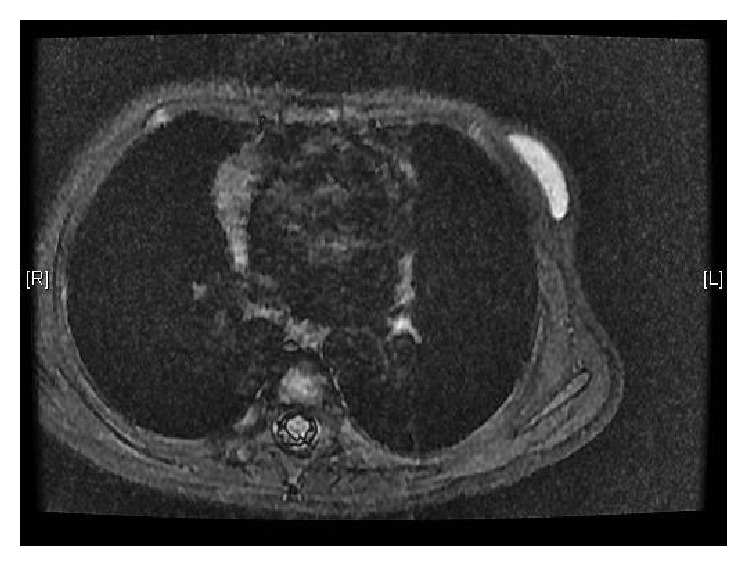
Magnetic resonance imaging showing a T1 hypertense lesion in left breast.
